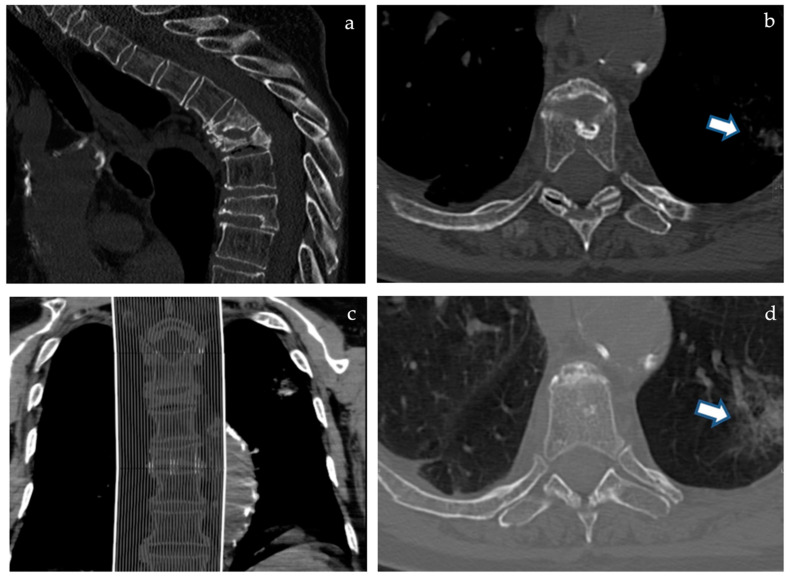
(a) (above left), (b) (above right), (c) (below left), and (d) (below right). A 77-year-old female patient with unbearable pain due to T5 and T6 spontaneous severe fractures (a,c) performed CT among a different institution due to the scarce compliance required for Magnetic Resonance. While reviewing CT imaging in our Interventional Radiology consultation office to assess the indication for vertebroplasty, a left lung opacity was noticed on “bone window” (b, arrow) and was confirmed and more evident when switching to “lung window” view mode (d, arrow).
